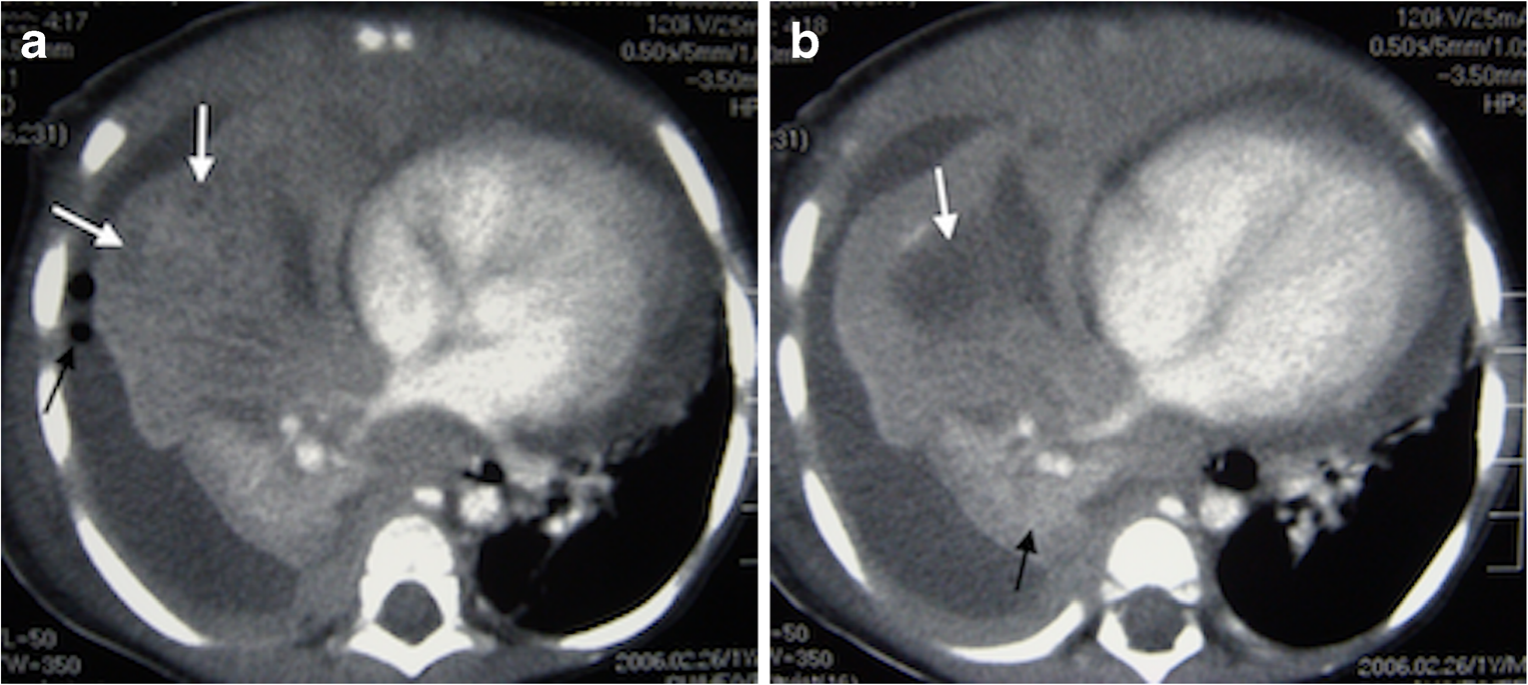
Representative axial contrast-enhanced CT of the chest in a 1-year-old boy with pneumonia, who was not responding to antibiotics. a Necrotising pneumonia in the right middle lobe is represented by low density, poorly/non-enhancing or liquefied areas of lung (*white arrows*). In addition, there is a right pleural effusion containing pockets of air *(black arrow)* resulting from an attempted drainage. b In contrast to the cavitary process in the centre of the necrotising lung (*white arrow*), the viable consolidated right lower lobe demonstrates enhancement (*black arrow*)
